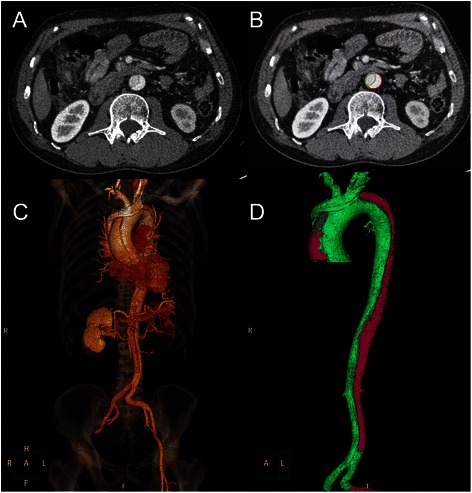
In each patient, we performed volumetric analysis of contrast-enhanced helical computed tomography (CT) covering the entire aorta starting from the innominate artery to the iliac bifurcation. Segmentations of the true lumen (TL, green) and false lumen (FL, red) were performed and volumes were calculated separately
